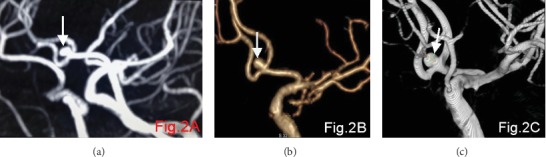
(a–c) show the MRA, CTA, and 3D-DSA, respectively, of the anterior communicating artery aneurysm in the same patient. The arrows show the aneurysm.
